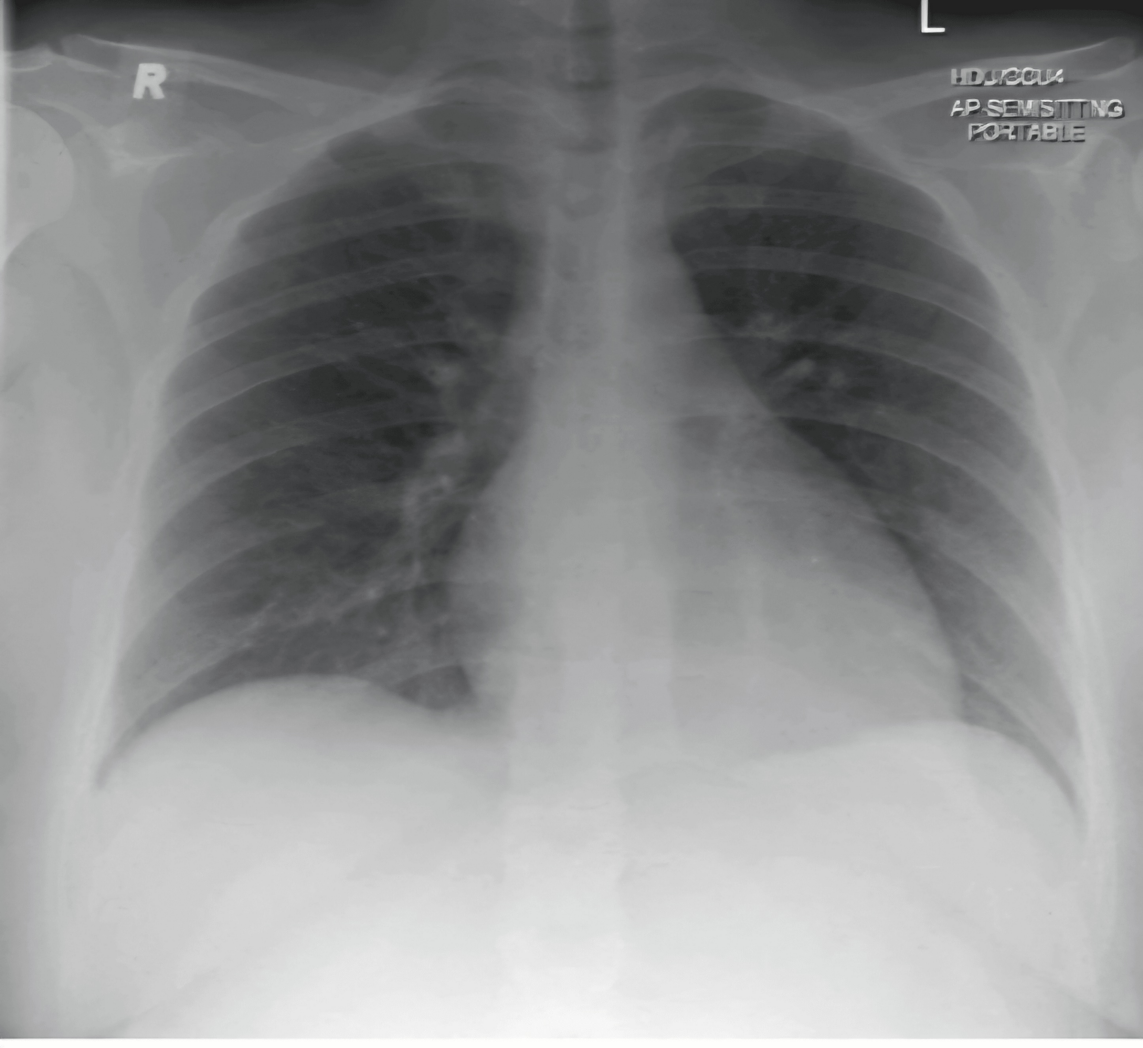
Repeat chest X-ray on day five showing resolution of the previously reported bronchopneumonia
